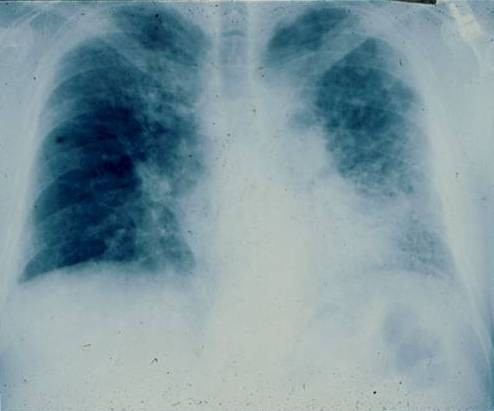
PA chest radiograph of a 67-year old man with progressive dyspnea revealing bilateral reticular infiltrates with lower lobe predominance. * Reprinted from Fishman's Pulmonary Diseases and Disorders, 4^th ^edition 2007. Meltzer, EB and Noble, PW: Chapter 70, Idiopathic Pulmonary Fibrosis. With permission from McGraw-Hill Companies.
